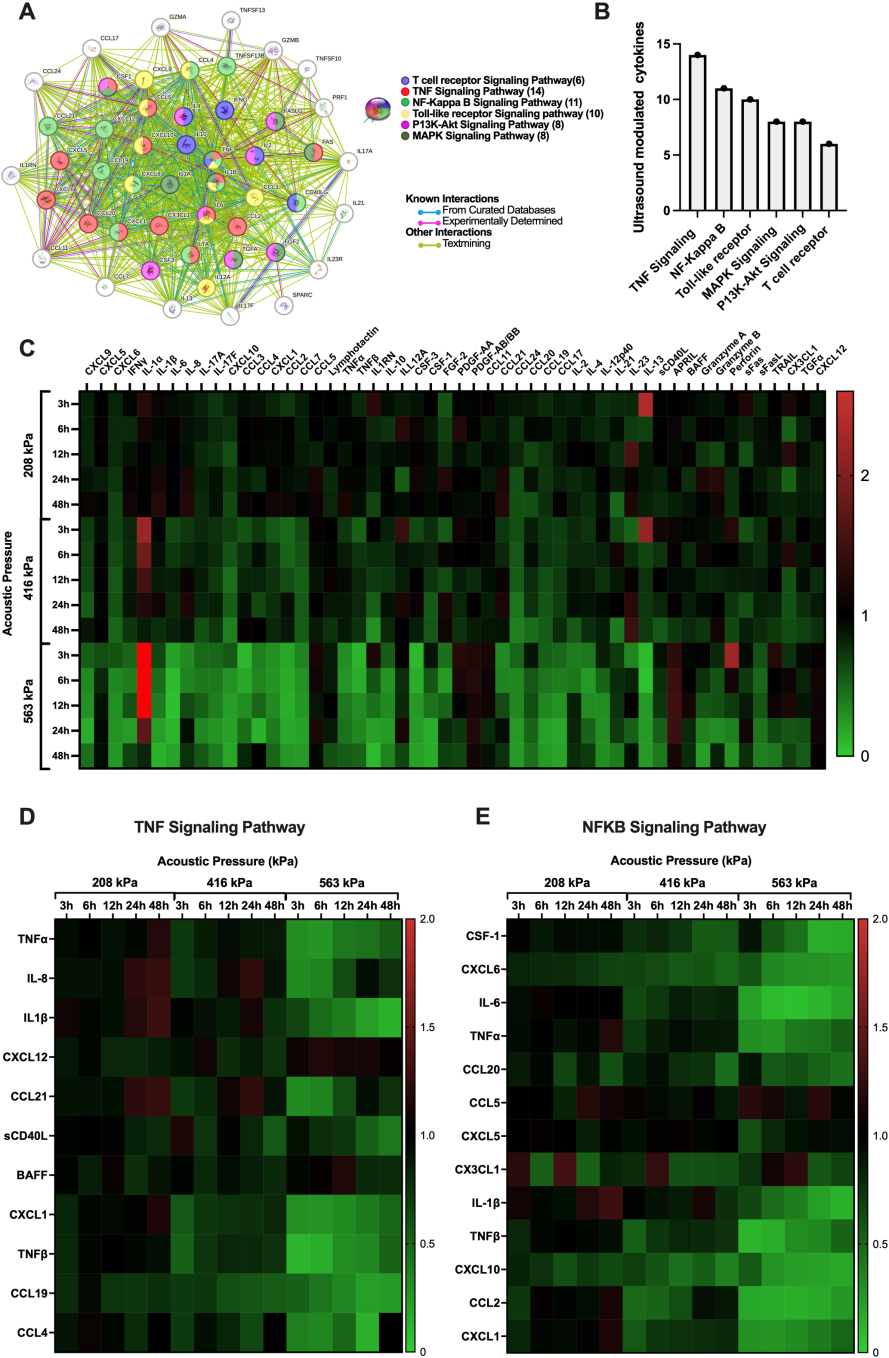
Microbubble-mediated treatment of immune cells elicits time-dependent changes to cytokine and other analyte secretion that are highly dependent on the transmit acoustic pressure. (A) String protein interaction map showing the functional links and pathways between analytes that were affected by ultrasound treatment as compartmentalized using the KEGG database collection, globally summarized via the (B) number of proteins that were modulated in a few major pathways related to cancer. Note that the TNF and NFκB pathways are the most affected by ultrasound, in terms of the absolute number of relevant analytes. All pathway connections here are characterized by false discovery rates *p*<0.001. See text for details. (C) Heatmap with all significantly modulated cytokine fold changes in PBMCs. (D) Heatmaps within the TNF and (E) the NFκB signaling pathway depict the effect and immunomodulation of microbubble-mediated focused ultrasound treatment on the secretome of PBMCs. Fold increase in cytokine production compared to sham controls is depicted as red and a decrease is depicted as green. Significance is determined as *p*<0.05.
